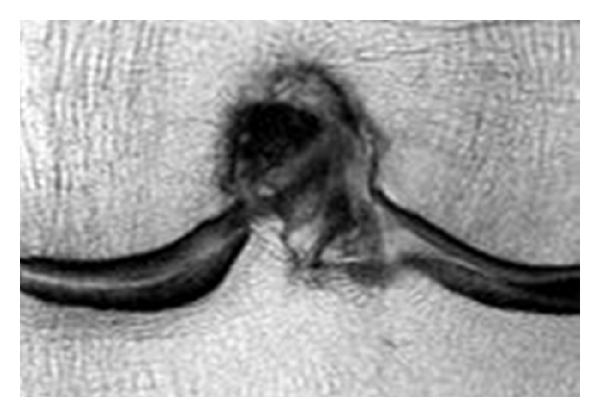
A sample MRI image used in this study.
